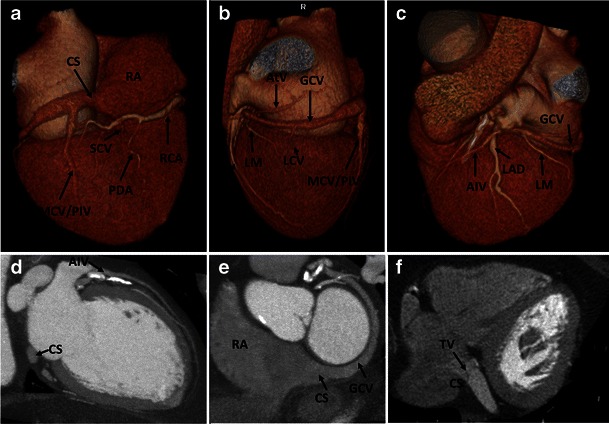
(a, b, c) The 3D volume-rendered and (d, e) multiplanar reformat CT images of the heart showing coronary venous anatomy. The CS is located in the posterior atrioventricular groove and opens into the right atrium via the CS ostium. It is a continuation of the great cardiac vein and collects blood from the tributary veins of both ventricles and atria. The main ventricular venous tributaries include the anterior interventricular vein, lateral cardiac vein, posterior ventricular vein (not shown), middle cardiac vein (also known as the posterior interventricular vein) and small cardiac vein. (f) Axial CCT images demonstrating the valve of Thebesius. RA, right atrium; CS, coronary sinus; GCV, great cardiac vein; SCV, small cardiac vein; MCV/PIV, middle cardiac vein/posterior interventricular vein; LCV, lateral cardiac vein; AIV, anterior interventricular vein; LAD, left anterior descending artery; LM, left marginal artery; RCA, right coronary artery; PDA, posterior descending artery; AtV, atrial vein; TV, valve of Thebesius
